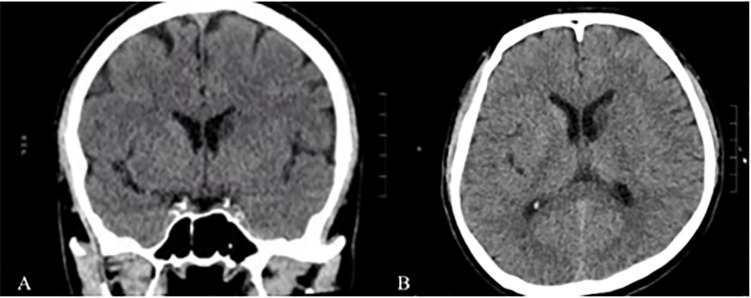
Coronal (A) and axial (B) brain computed tomography representative images of a 58-year-old male presenting with a headache, hand numbness, and phantosmia.
